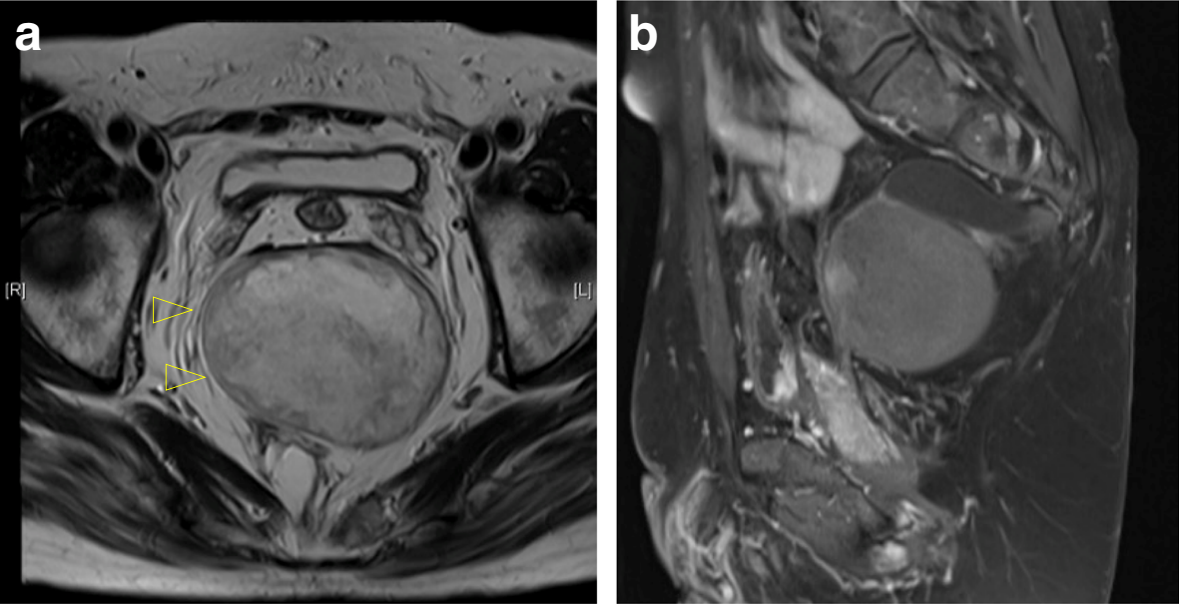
Magnetic resonance imaging (MRI). The inside of the cystic tumor (△) showed heterogeneously high intensity in T2-weighted image (a) and homogenously low intensity in T1-weighted image. The wall was enhanced by Gadolinium (b)
